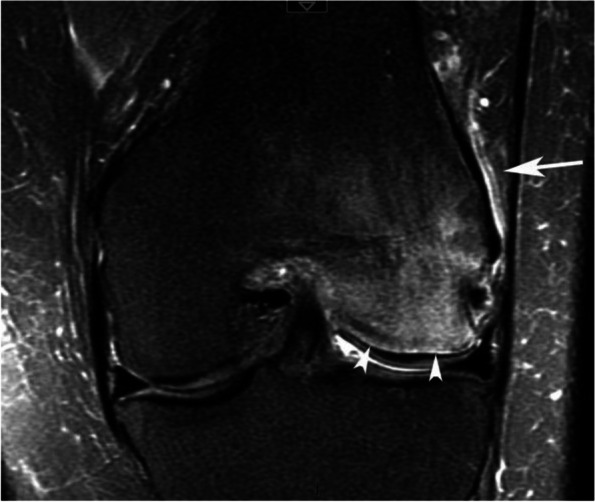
Other features associated with bone-marrow-like signal. In case of intense BME-like signal intensity, the area immediately adjacent to the subchondral bone plate may show a very intense, finely linear high signal on fat-suppressed T2-weighted images (arrowheads), which could be linked to hyperemia of this richly vascularized area. Edema-like high signal intensity may also involve the neighboring soft tissues (arrow)
